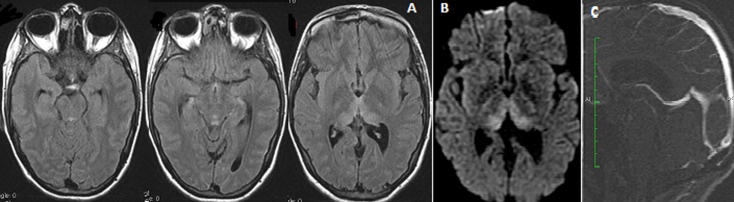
IRM cérébrale axiale FLAIR (A), diffusion (B) montrant des lésions en hypersignal bilatérales et symétriques de la région paramédiane des thalami, periacqueducale et des tubercules mamillaires. L’angio-MR veineuse (C) est normale
